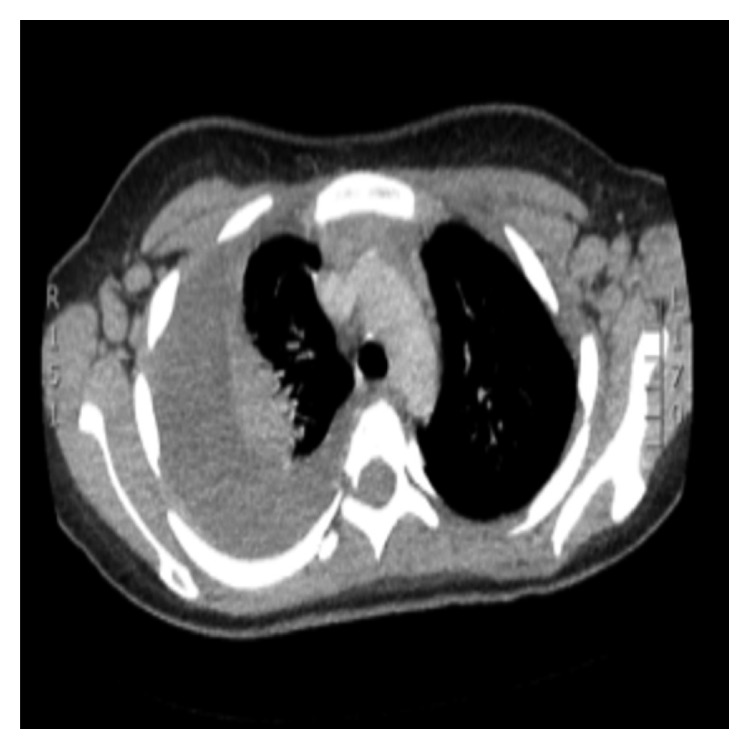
Contrast-enhanced CT thorax: large loculated right-sided pleural effusion. Small left-sided effusion. Bilateral axillary lymphadenopathy. Bulky left hilum.
